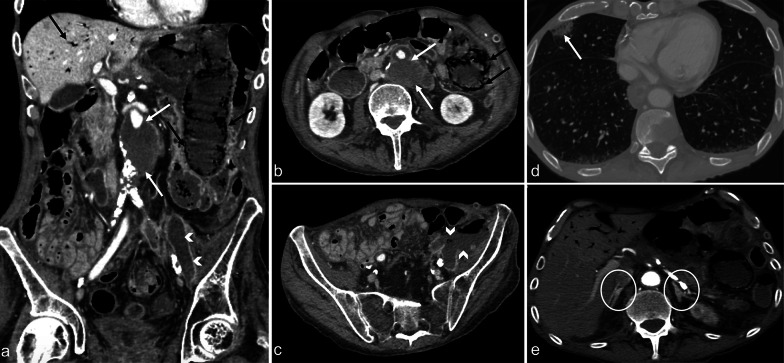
Contrast-enhanced CT images in the arterial phase showing a 75-year-old male with sepsis (qSOFA 3) due to an infected aneurysm after aorto-basilic stent placement (a and b, white arrows). Concomitant intestinal images reveal liver septic pneumatosis (a and b, black arrows), stercoraceous collection in the left iliac fossa (c, white arrowheads), and septic emboli with pulmonary infarction (d, white arrows). The adrenal glands display hyperenhancement (e, white circles)
